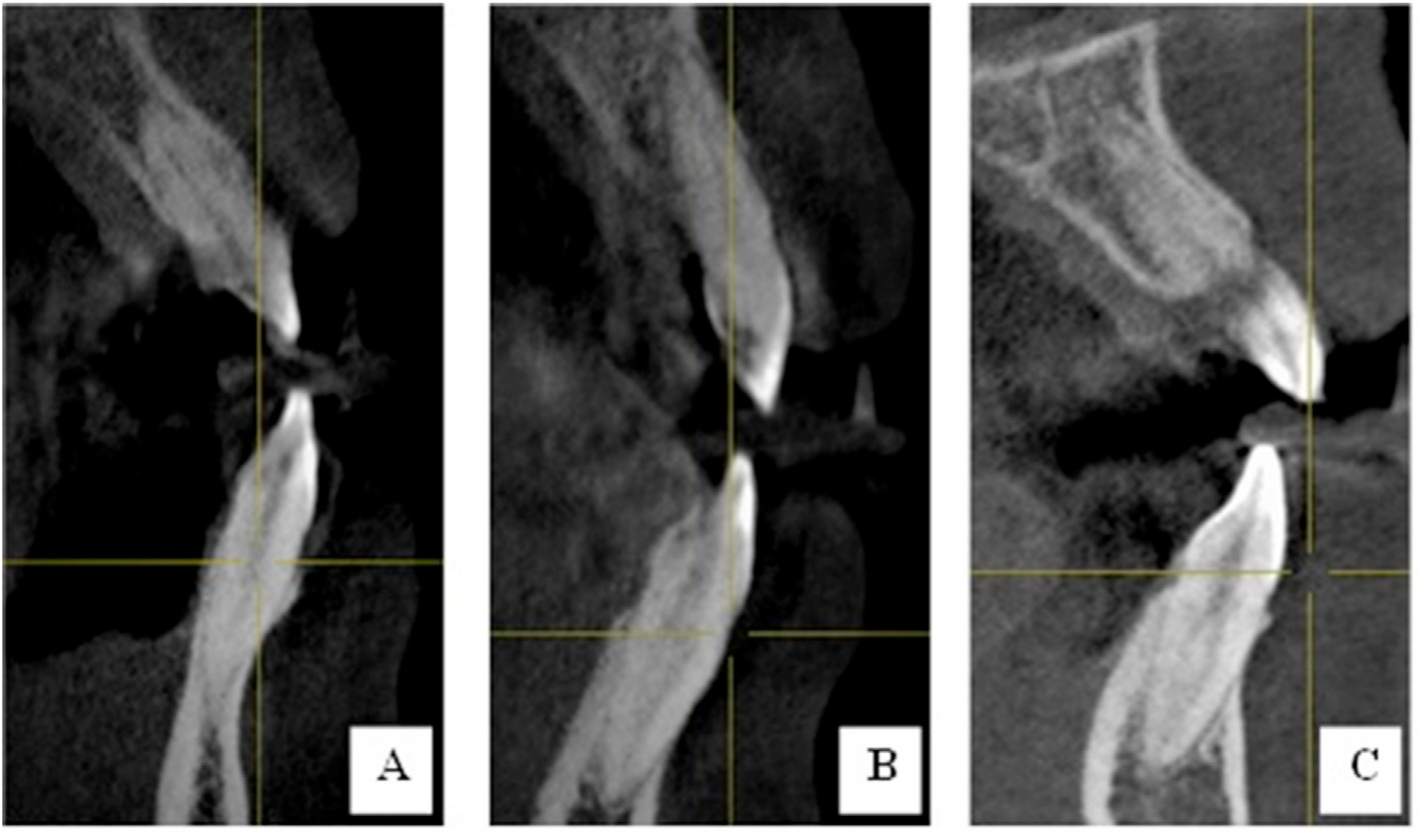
CBCT coronal section of mandibular lateral incisor (A: Type I; B: Type II; and Type III Vertucci's classification).
